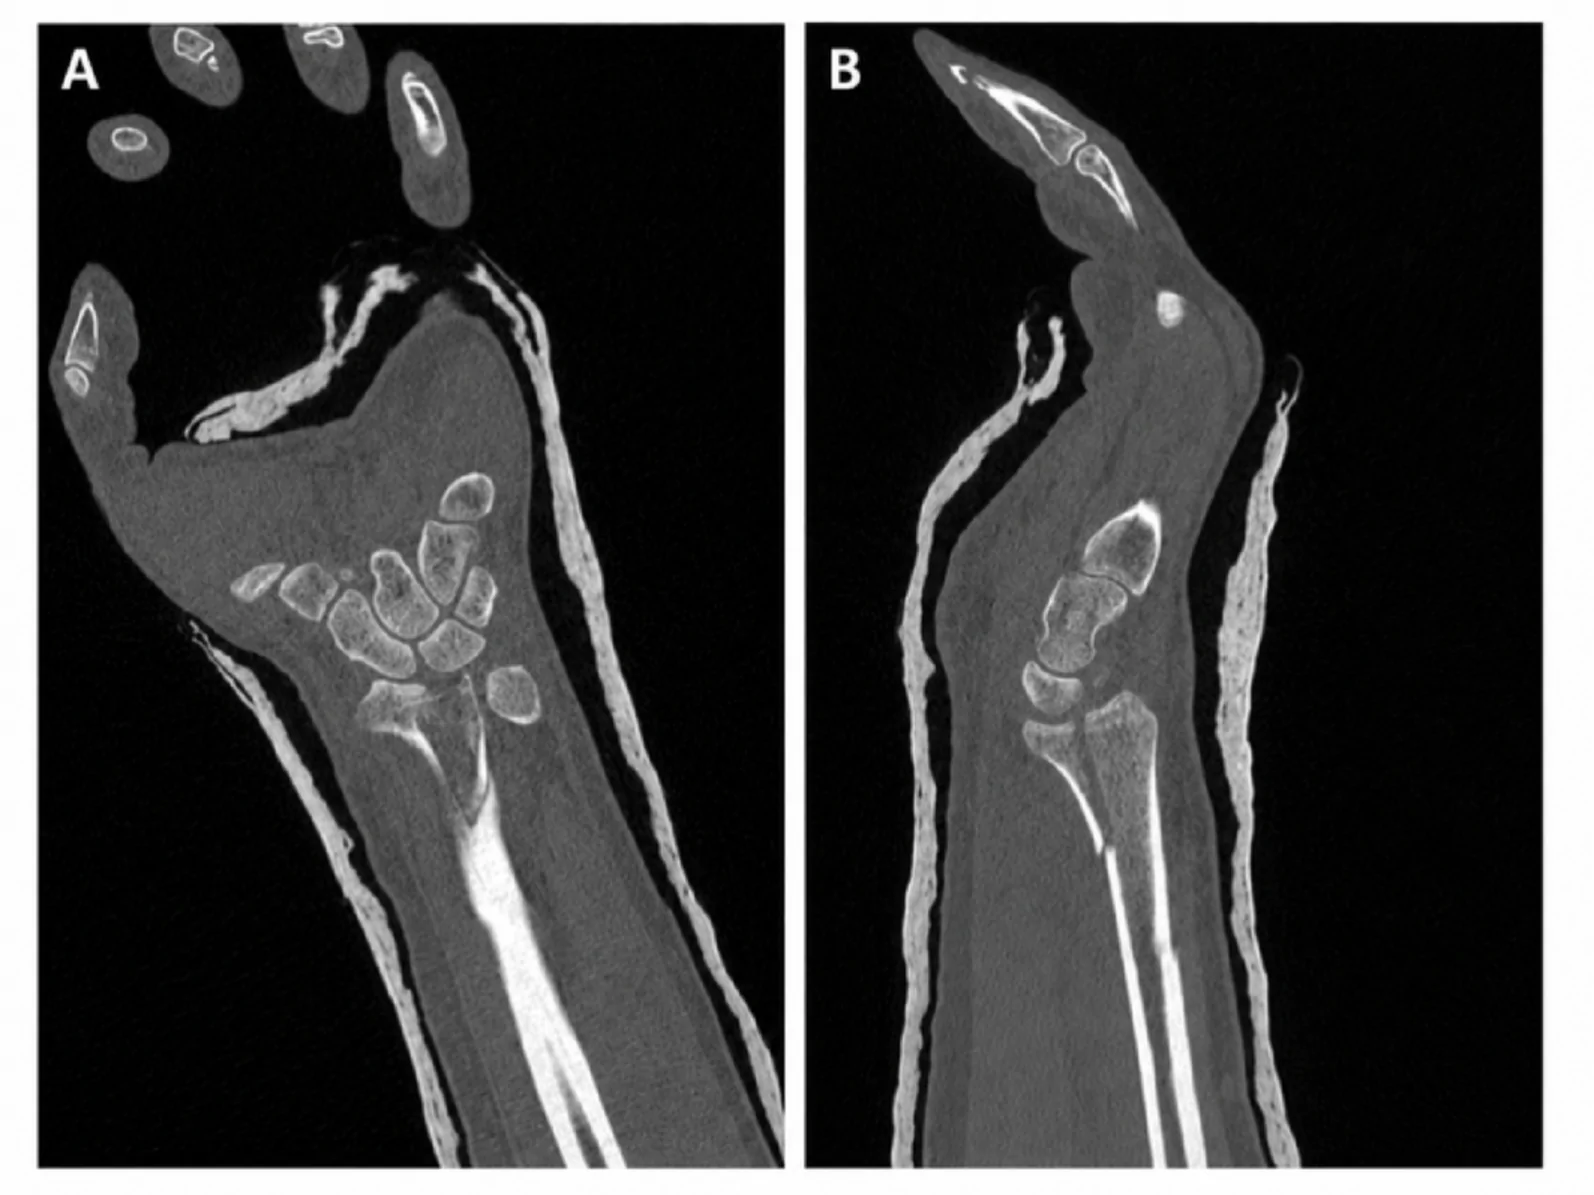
Representative 2D CT sections selected from the post-reduction GIF sequence of an AO Type B distal radius fracture. A Coronal reconstructed section. B Sagittal reconstructed section
